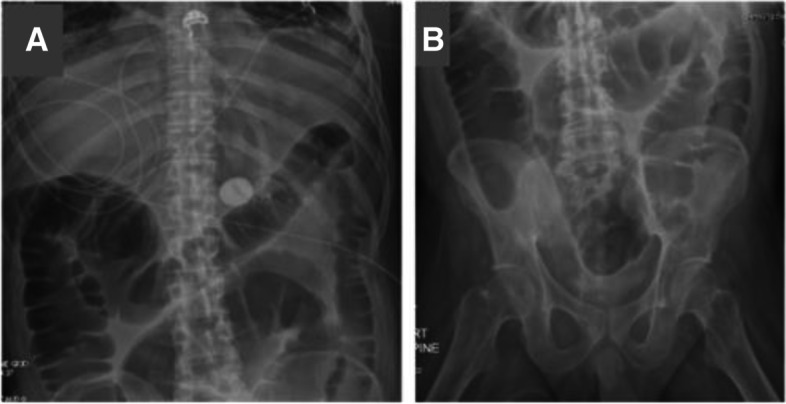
Abdominal X-ray documenting the course of the ileus. A, B Abdominal x-rays showing dilated large intestine bowel loops without any discernable obstructions
